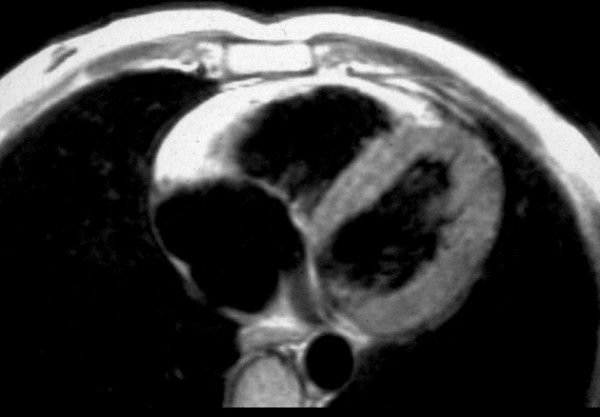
MRI in a patient affected by ARVC/D (long axis view of the right ventricle): note the transmural diffuse bright signal in the RV free wall on spin echo T1 (a) due to massive myocardial atrophy with fatty replacement (b).
